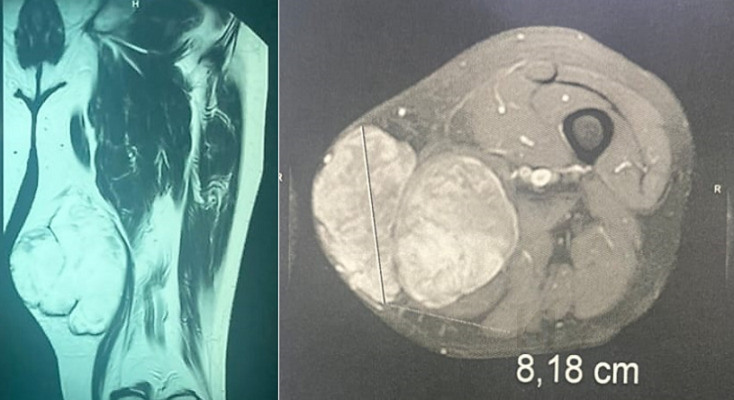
MRI of the thigh showing a well limited soft tissue mass, roughly rounded, with polylobed contours, measuring 8cm in great axis, pushing the medial rectus muscle, respecting the femur and the vascular-nervous bundle
